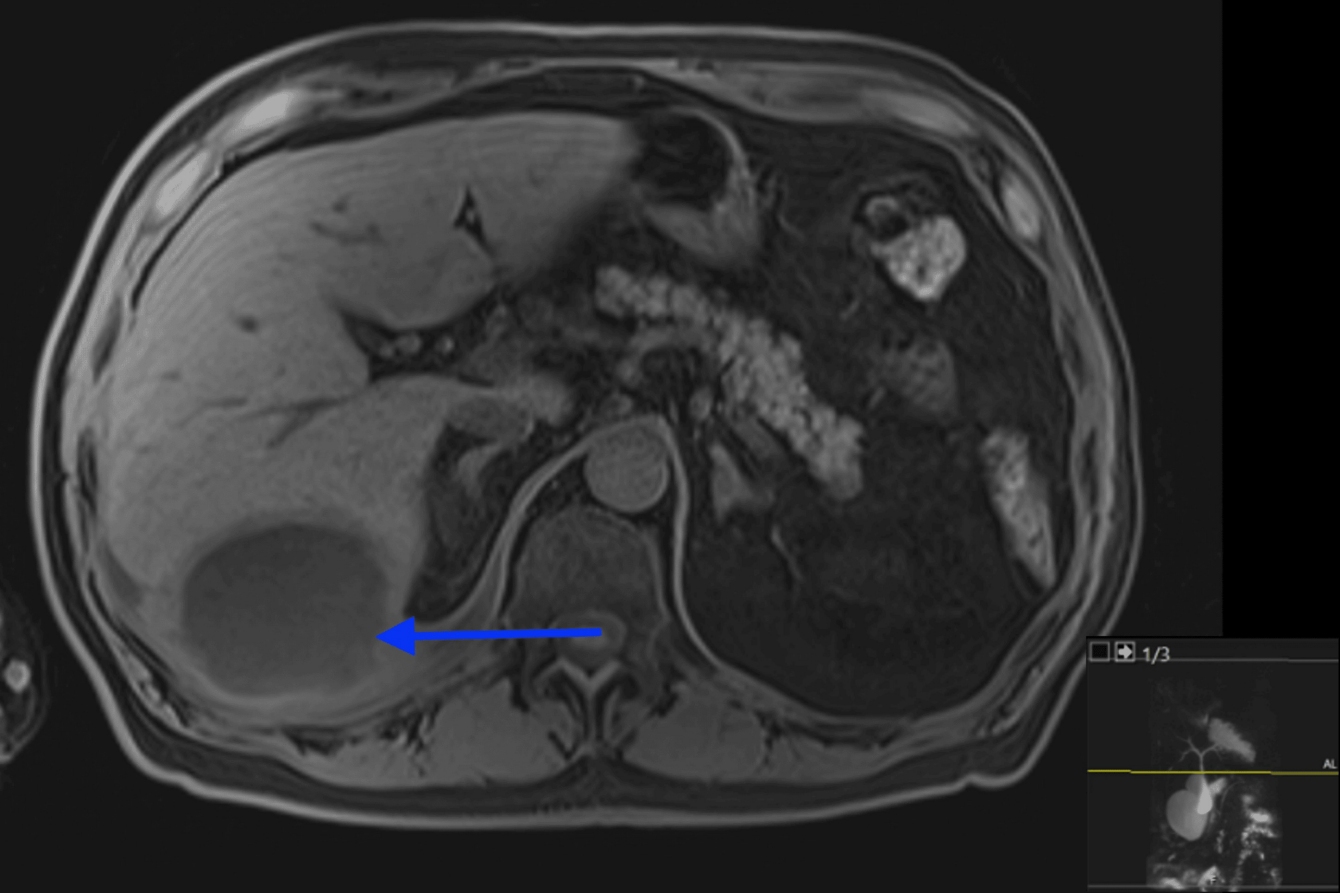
Contrast-enhanced MRI of the abdomen showing a rim-enhancing fluid collection posterior to the right hepatic lobe. The peripheral enhancement and adjacent hepatic capsule involvement are consistent with a localized subhepatic abscess.
